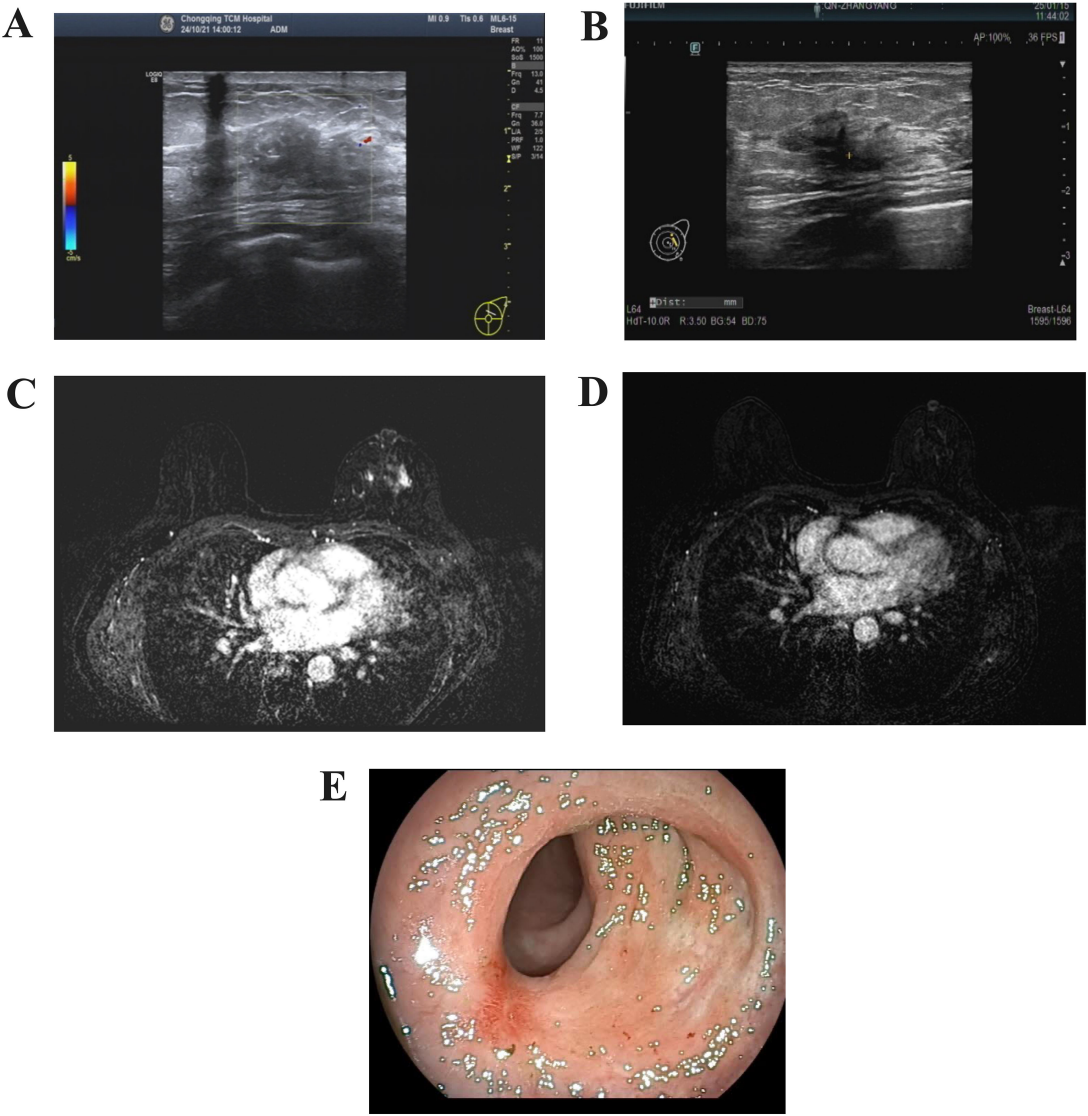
Post-treatment tumor imaging. (A) Color Doppler ultrasound of the breast(two cycles of HP-targeted therapy). (B) Color Doppler ultrasound of the breast(six cycles of HP-targeted therapy). (C) Contrast-enhanced MRI scan of the breast(two cycles of HP-targeted therapy). (D) Contrast-enhanced MRI scan of the breast(six cycles of HP-targeted therapy). (E) Endoscopic appearance of the rectal lesion after treatment.
